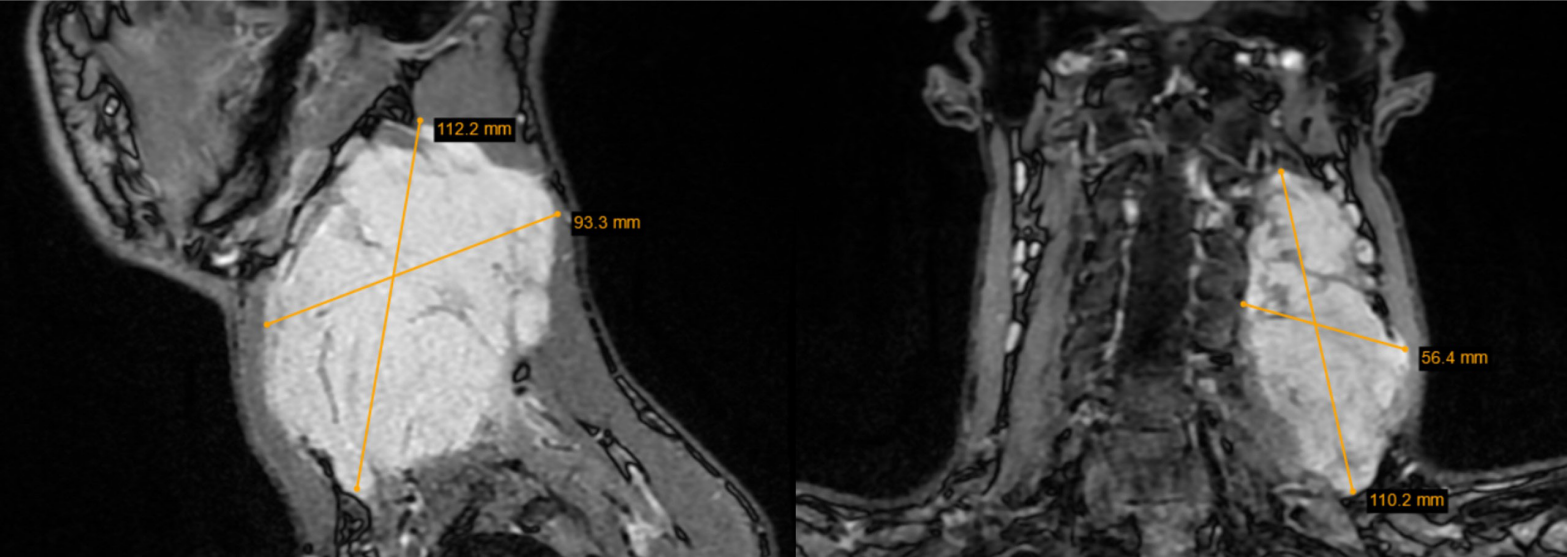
Magnetic resonance imaging (left—sagittal view; right—coronal view) of a recurrent tumor of the perivertebral space measuring 110 × 56 × 93 mm.
